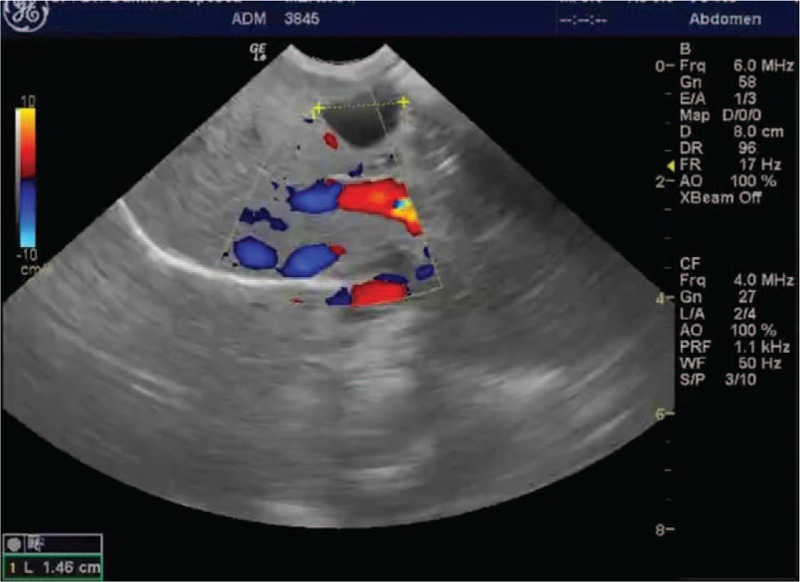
Transabdominal color Doppler ultrasound immediately after delivery (using a convex probe).
